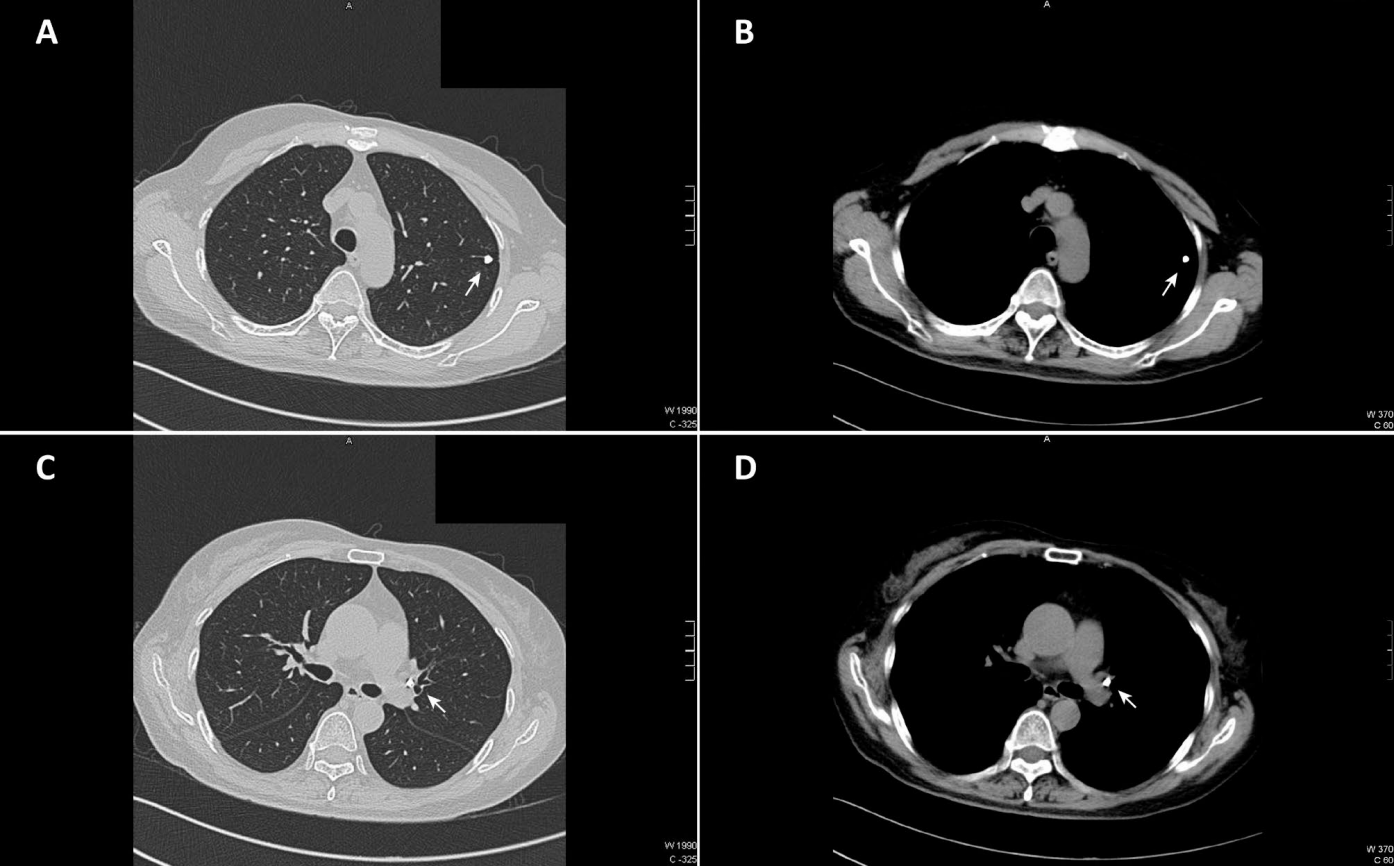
Chest CT findings. There were no overt imaging abnormalities. Only sporadic calcifications were found in the left lung (A, B; white arrows) and near left hilar regions (C, D; white arrows)
